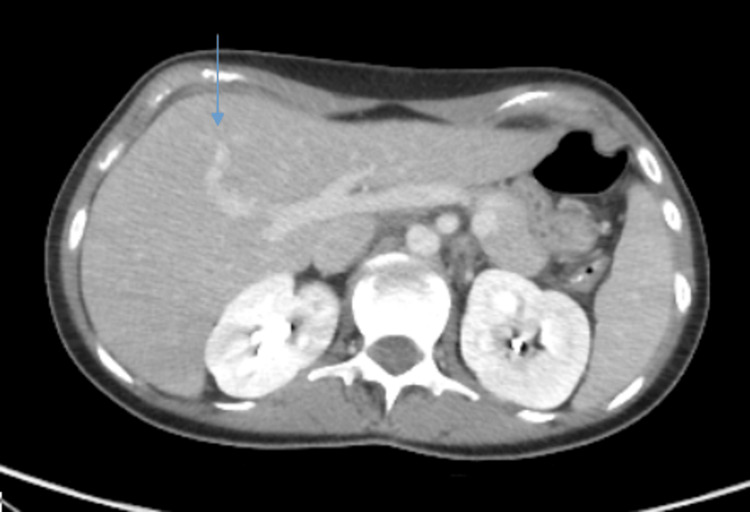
CT scan of the abdomen showing enlarged liver
